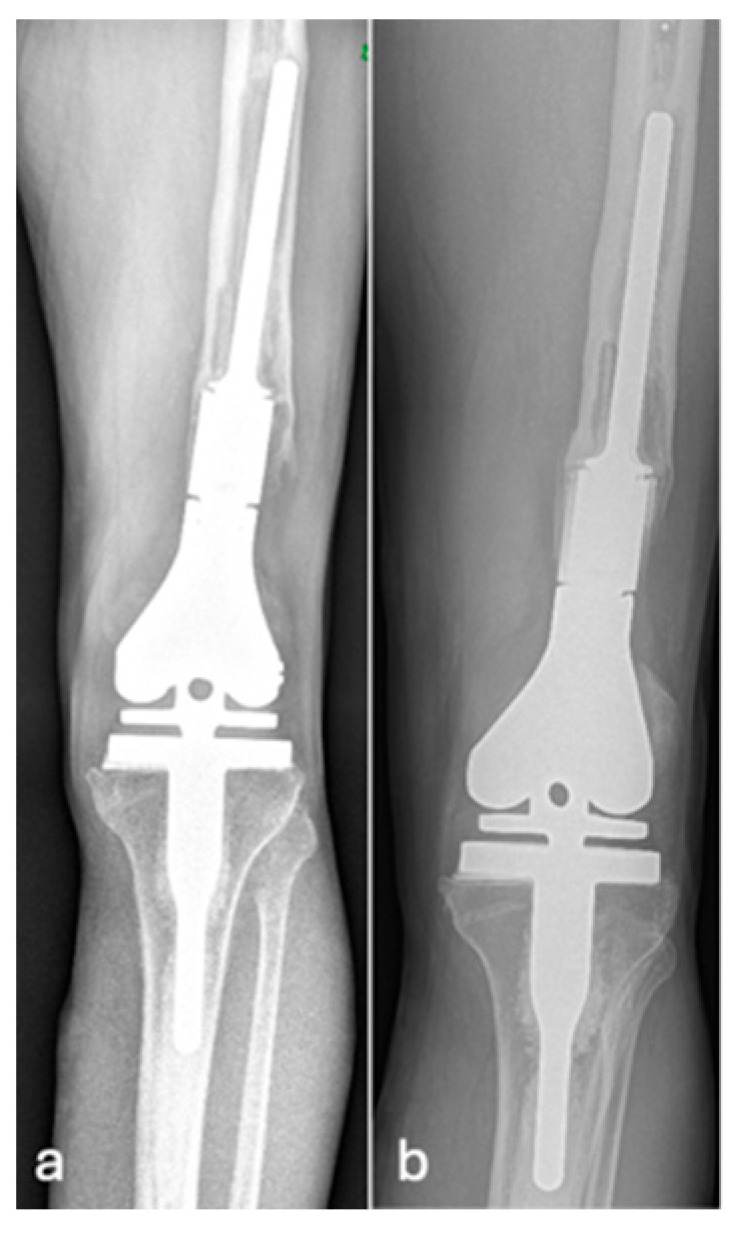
AP radiographs (a,b) showing grade 3 loosening of EPR, loosening around the peg of femoral component.
